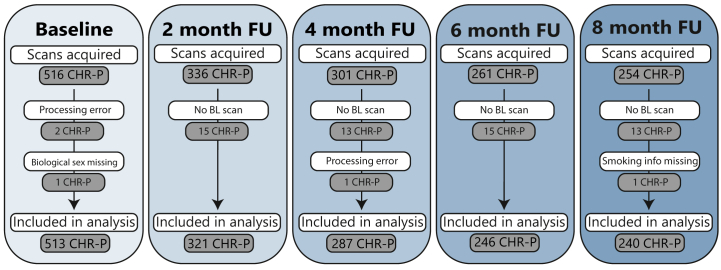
Number of participants and reasons for exclusion from the analysis. Scans of participants without baseline magnetic resonance imaging data were excluded (*n* = 15 at month [M] 2, *n* = 13 at M4, *n* = 15 at M6, *n* = 13 at M8). Moreover, we excluded participants with no information on smoking behavior (*n* = 1 clinical high risk for psychosis [CHR-P] at M8) and no information on sex assigned at birth (*n* = 1 CHR-P at baseline). Additionally, 3 participants were excluded due to preprocessing errors (*n* = 2 CHR-P at baseline, *n* = 1 CHR-P at M4). FU, follow-up.
